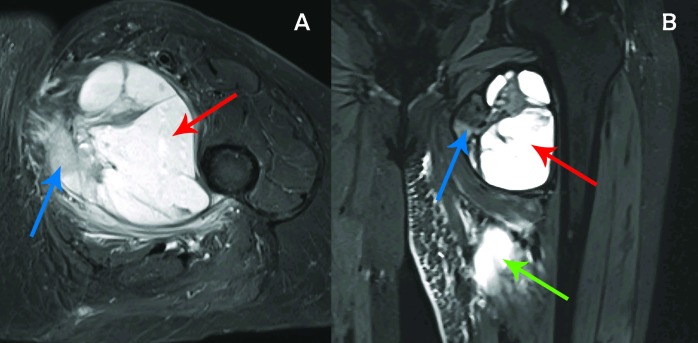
Mid-treatment magnetic resonance imaging Mid-treatment MRI (T1 TIRM) revealing interval growth in the mass now measuring 8.2 cm x 8.5 cm x 9.5 cm in the axial (A) and coronal (B) views. Note the region of large cystic necrosis (red arrow) and soft tissue encapsulated component (blue arrow). Also, note the increased muscular edema (green arrow) which extends inferiorly to below midshaft of femur. MRI: magnetic resonance imaging; TIRM: turbo inversion recovery magnitude
